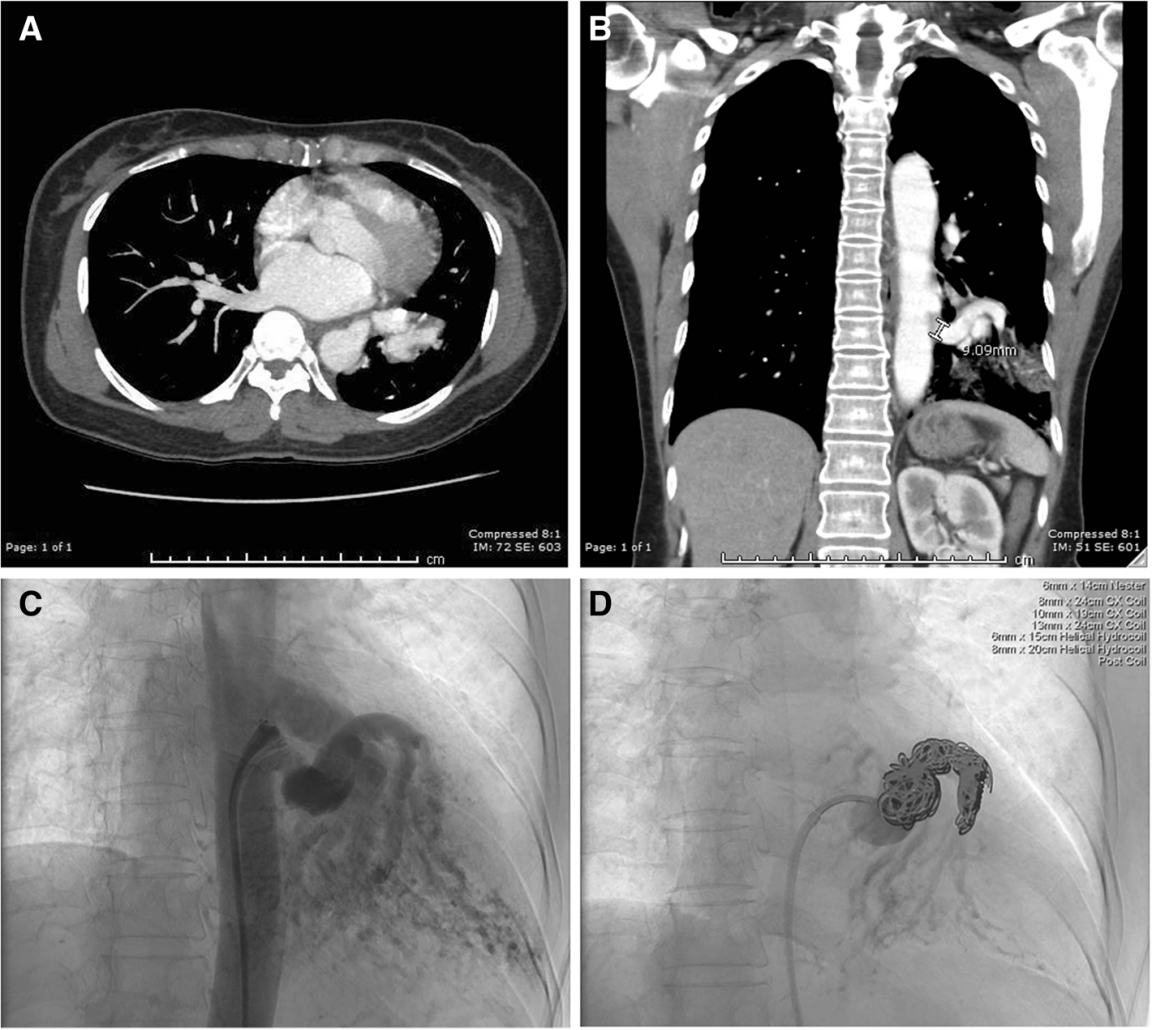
a Axial computed tomographic scan with contrast showing a tortuous vessel that leads to a focal area of mixed consolidation and ground glass representing intralobar pulmonary sequestration. b Coronal view showing the origin of the aberrant vessel, which measures approximately 1 cm. c Angiography of vessel prior to embolization. d Postembolization angiogram showing coils in place with no considerable flow
